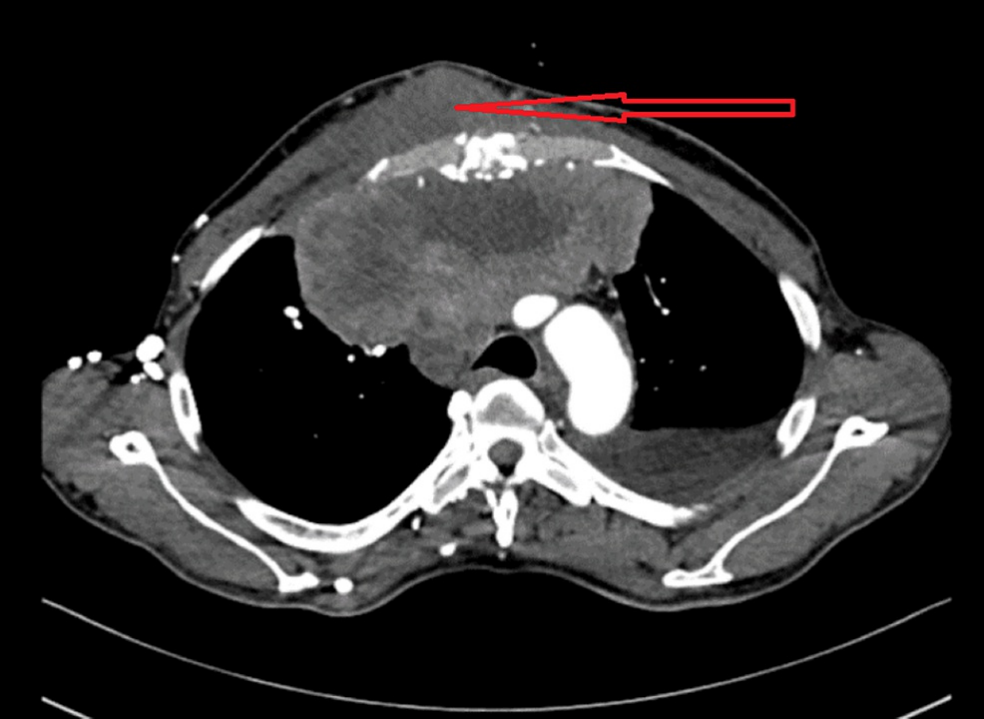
Contrast-enhanced CT thorax showing anterior mediastinal mass infiltrating the chest wall.
